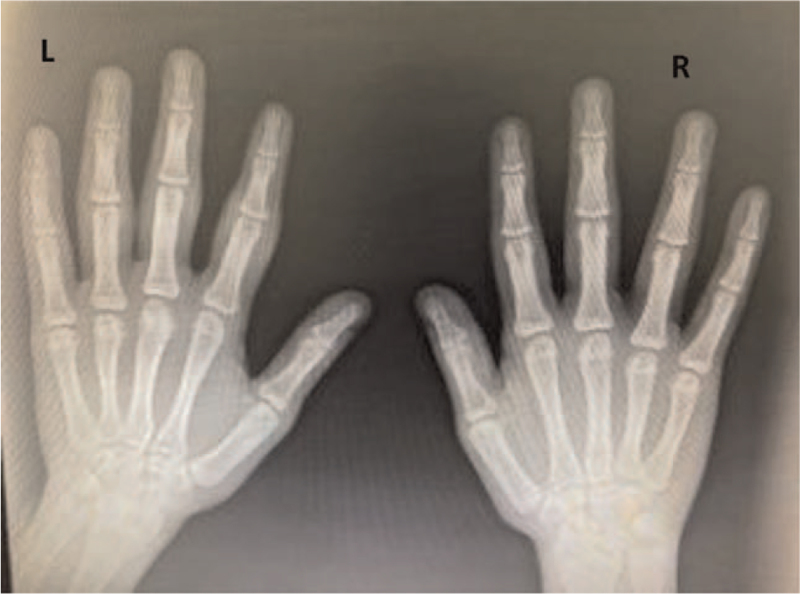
Plain radiograph of both hands showing soft tissue swelling of the second, third, and fourth digits of the left hand with no bone or joint involvement.
